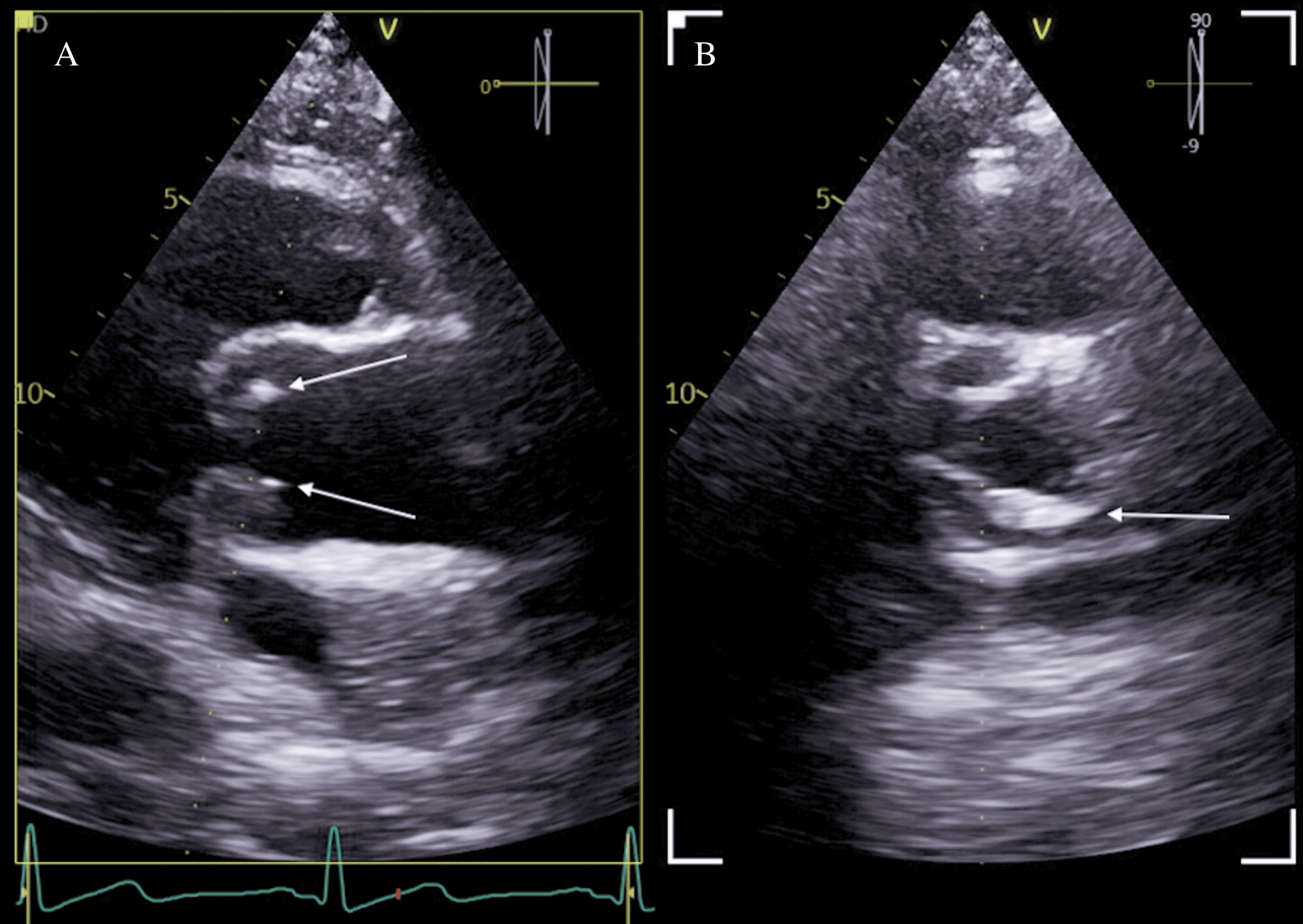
Transthoracic echocardiogram (TTE) findings Arrows in A and B point to a small mobile structure on the aortic valve, but are not suggestive of vegetation.
